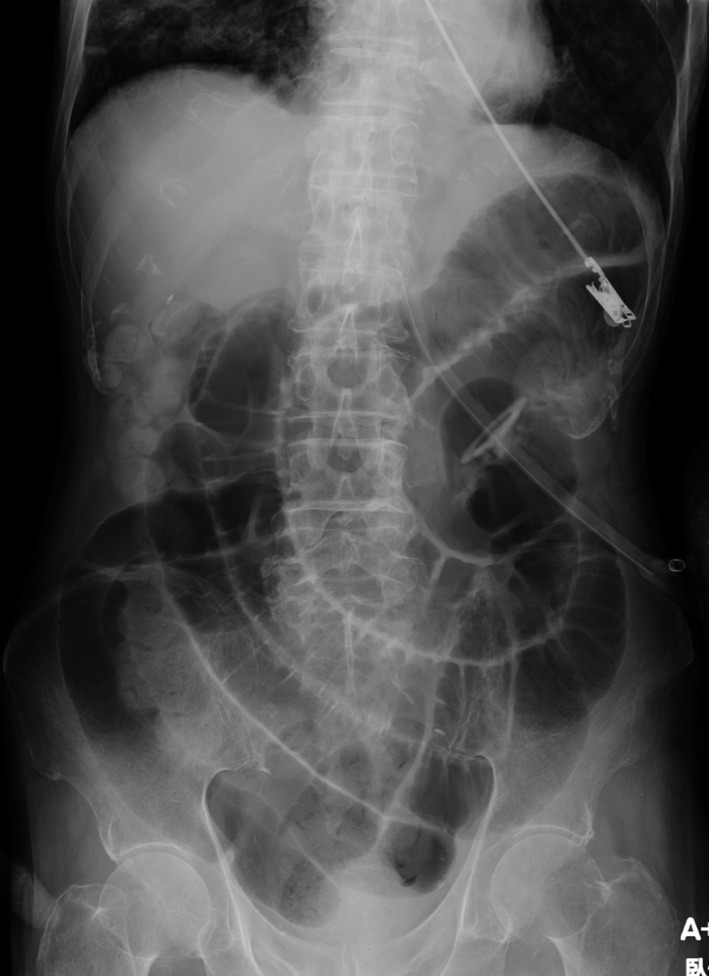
Abdominal radiograph revealing marked dilation of the small bowel due to adhesive ileus.
